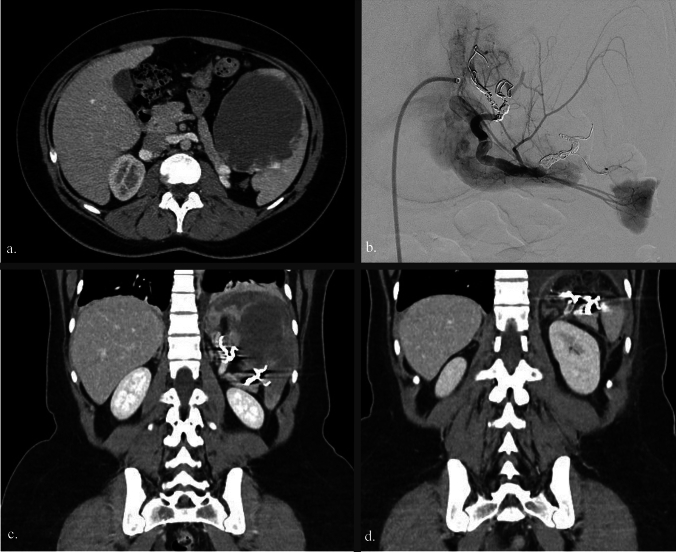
Preprocedural computed tomography (CT) scan (a); embolization of two branches of the splenic artery (b); post-embolization CT scan (c); postoperative CT scan (d)
